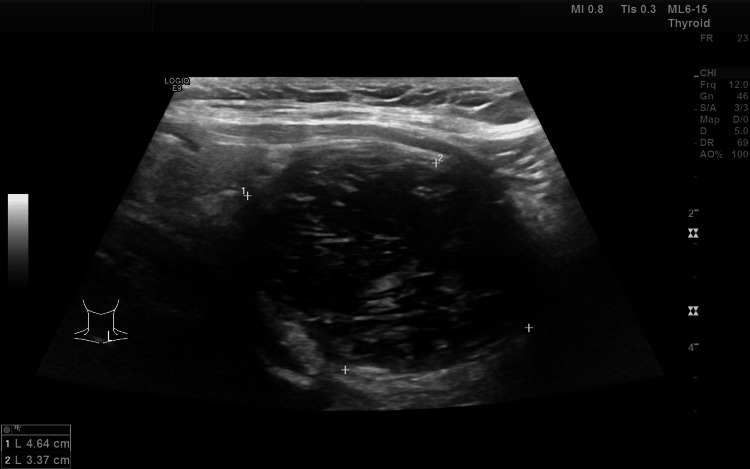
Ultrasound (US) image of the thyroid gland showing an exophytic nodule measuring 4.6 x 3.4 cm, having a solid and cystic component with a spongiform appearance arising from the lower pole of the left lobe of the thyroid gland.
